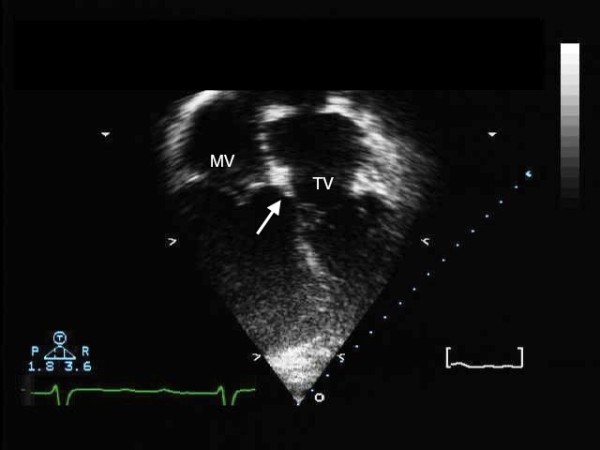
The four-chamber transthoracic echocardiogram shows reversed off-setting of the leaflets of the mitral and tricuspid valves.
